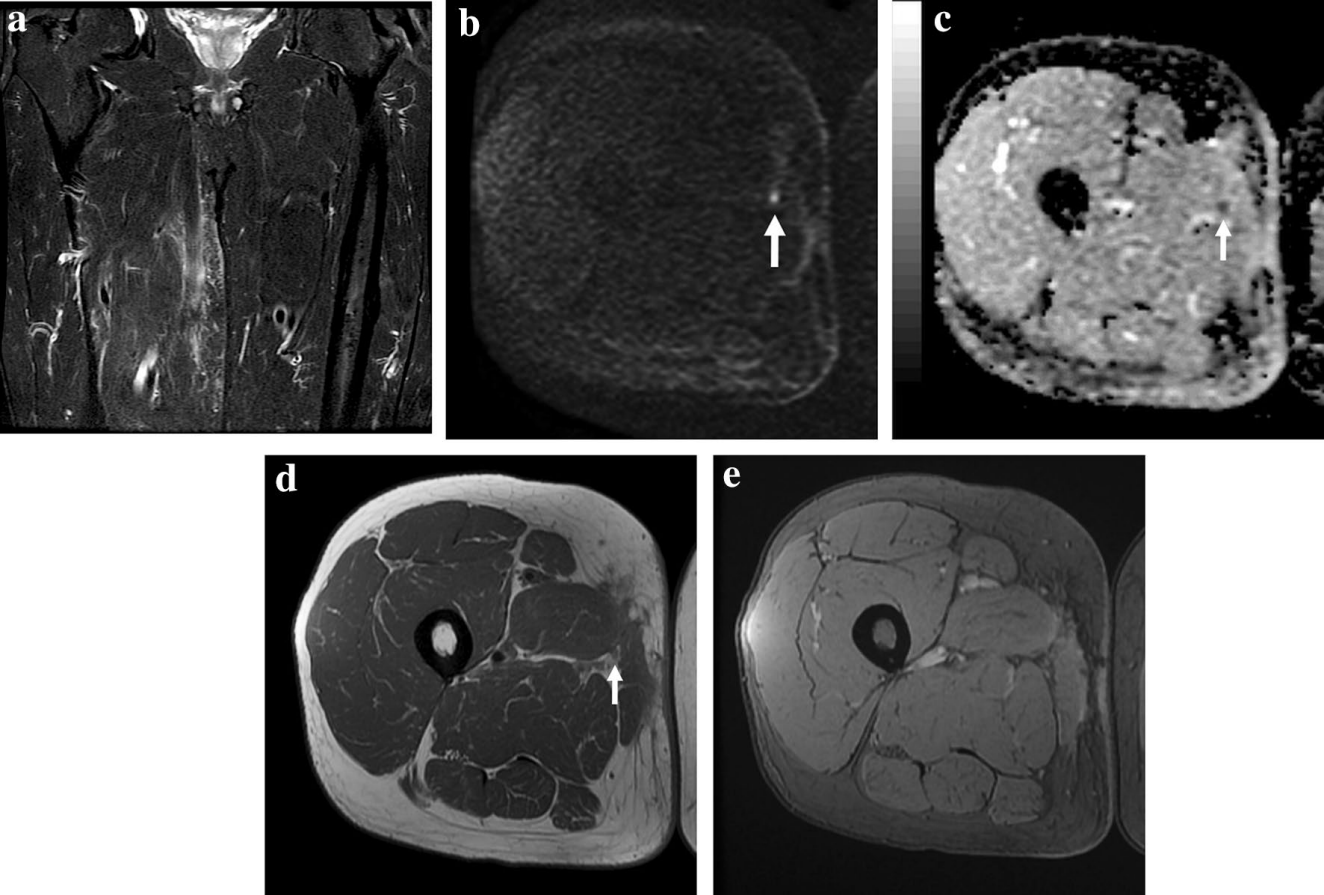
False positive case in which DWI findings were misinterpreted as small residual tumoral nodule. Fifty-three-year-old man MR follow-up after surgical excision of liposarcoma of the right thigh with positive margins. a Coronal STIR image shows diffuse soft tissue post-surgical edema of the medial proximal thigh compartment. b Axial DWI reveals a small hyperintense nodularity (*arrow*) within the scar, corresponding to a hypointense focal lesion on c ADC map (ADC = 0.8 × 10^–9^ m^2^/s). d Axial PDw image reveals a tiny nodule (*arrow*) within the intermuscular fat tissue corresponding to the DWI finding. e Axial T2*w GRE image at the same level shows no ferromagnetic artifacts
